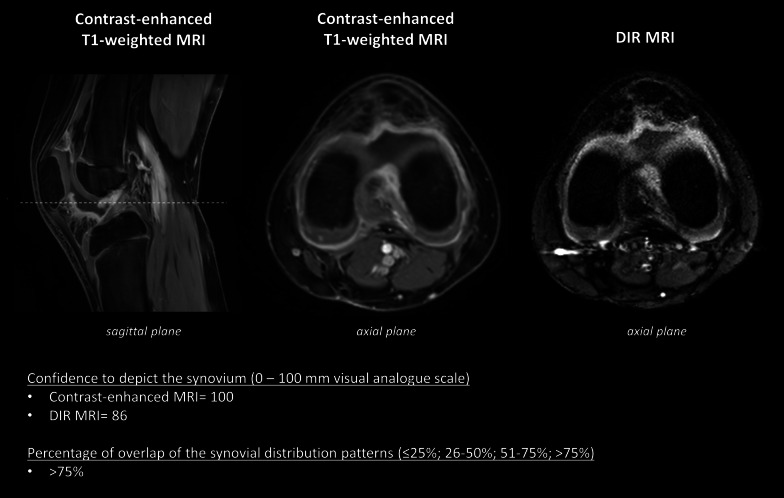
Images obtained in a 9-year-old girl, diagnosed with persistent oligoarticular JIA, with knee arthritis. Confidence to depict the synovium was 100 (0–100 mm visual analogue scale) for the axial contrast-enhanced MRI and 86 (0–100 mm visual analogue scale) for the axial DIR MRI. The percentage of overlap of synovial distribution patterns was scored as > 75%
